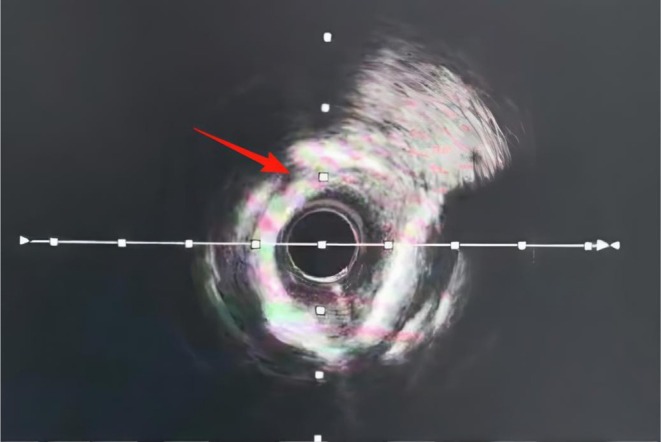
Destruction of calcified plaque after intravascular lithotripsy, as visualized on intravascular ultrasound.
